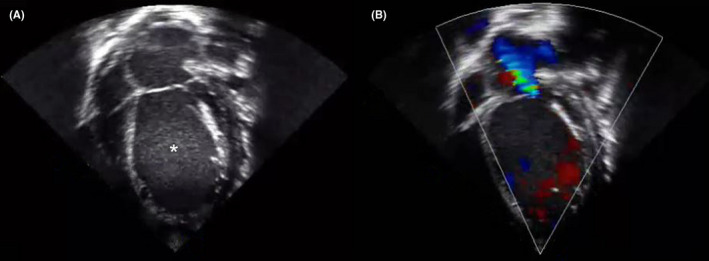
Postoperative transthoracic echocardiography. A, apical 4‐chamber view reveals left ventricular enlargement (asterisk). B, apical 4‐chamber view with doppler mode represents severe mitral regurgitation
